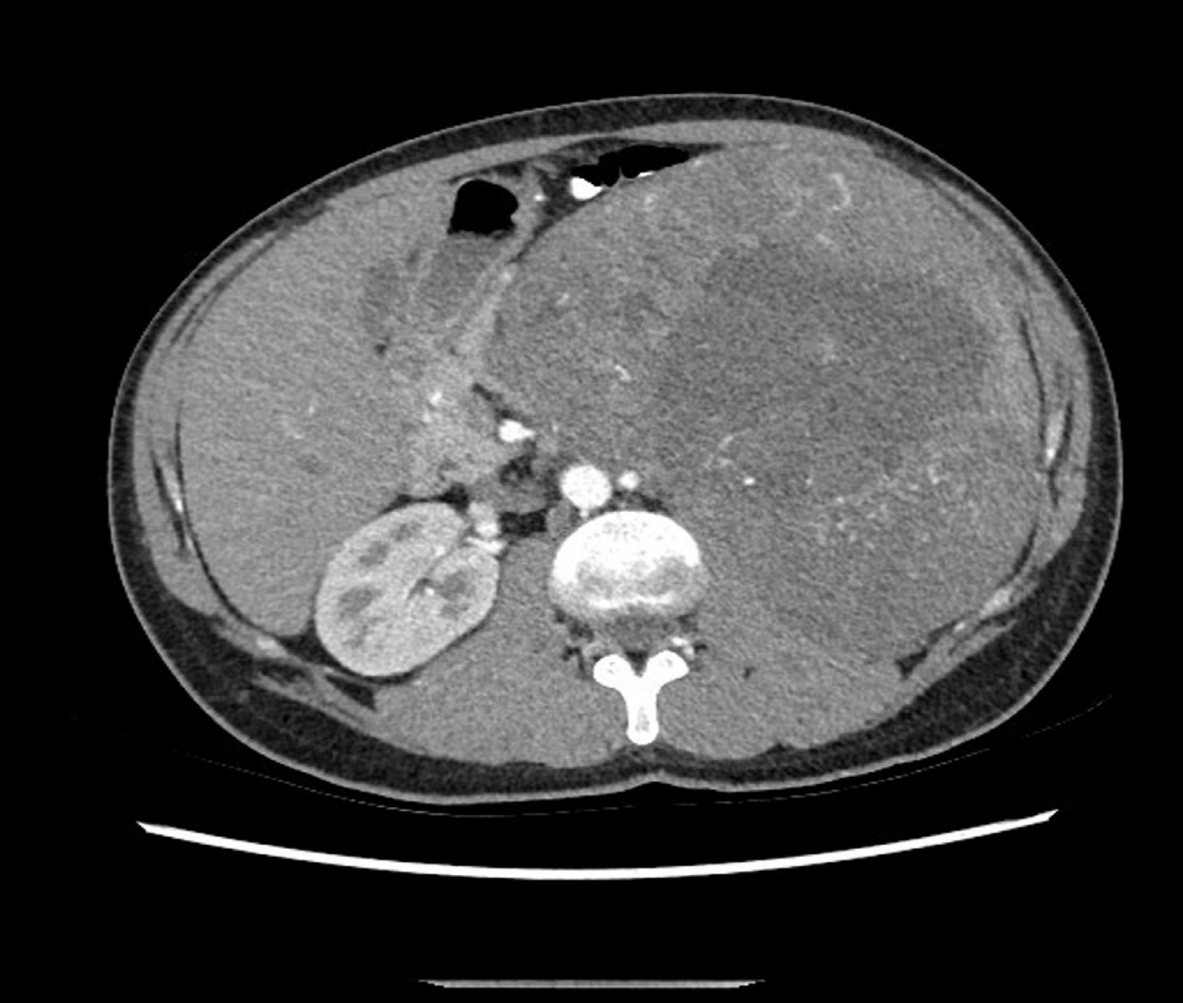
High resolution diagnostic Computed Tomography (HRCT) showed a large isodense mass in the left upper abdomen with areas of hypo-attenuation inside
